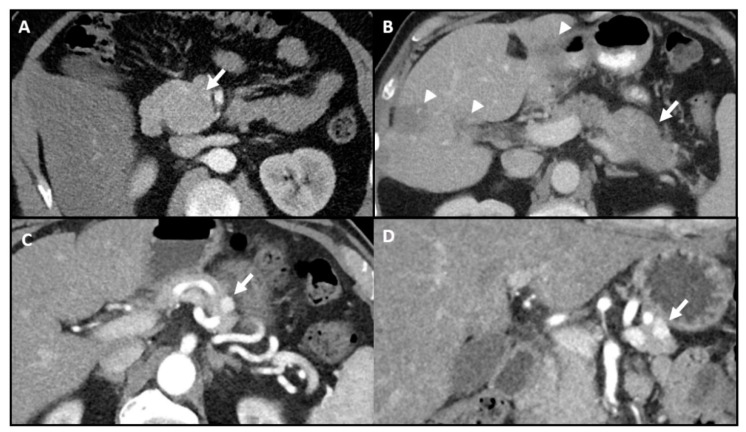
Variable appearance of pancreatic insulinomas on contrast-enhanced computed tomography (CT). (A) Axial image shows a 5.5 cm isodense insulinoma (arrow) in the uncinate process. (B) Axial image shows hypodense, malignant insulinoma within the pancreatic tail and liver metastases (arrowheads). (C,D) Axial and coronal images show an avidly enhancing 1.2 cm insulinoma in the pancreatic tail. All were proven to be pancreatic insulinomas by surgery or biopsy.
